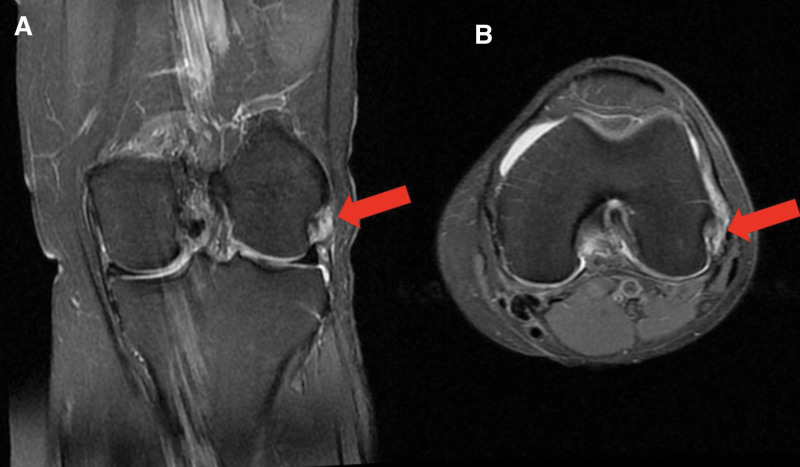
Coronal (A) and axial (B) MRI cuts demonstrating rupture of the anterolateral ligament at its femoral origin (red arrows). MRI = magnetic resonance imaging.
